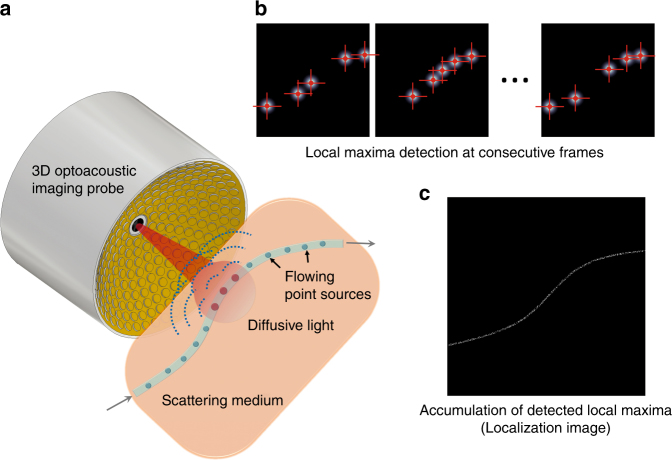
Imaging principles of localization optoacoustic tomography. a A spherical array of ultrasound transducers is used to acquire a three-dimensional optoacoustic image of flowing absorbers in an optical scattering medium for each laser pulse. b The positions of sparsely distributed absorbers are measured (localized) in a sequence of images. c An image is formed by superimposing the localized positions
